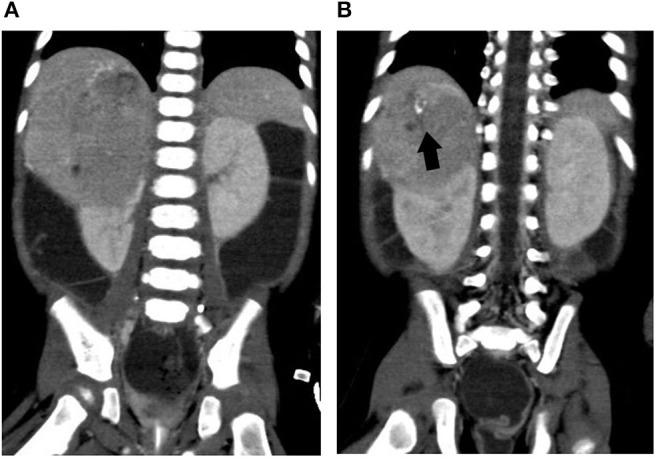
Abdominal computed tomography of case 2. (A) A huge right suprarenal mass lesion causing mass effect to adjacent liver and kidney. (B) A closer view of the part with calcification. Arrow (black), calcification in the tumor mass.
